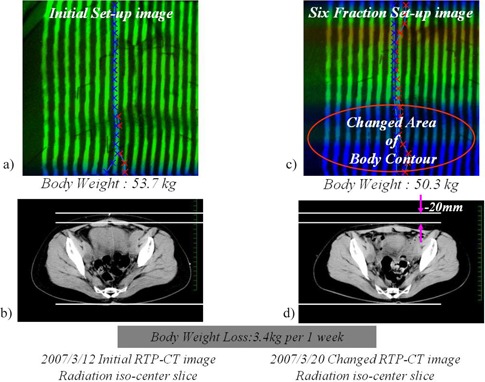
Clinical experience of Navi‐system: a) image at initial setup; b) CT scan at initial setup; c) image at the body weight loss of 3.4 kg; d) CT scan at the body weight loss of 3.4 kg.
